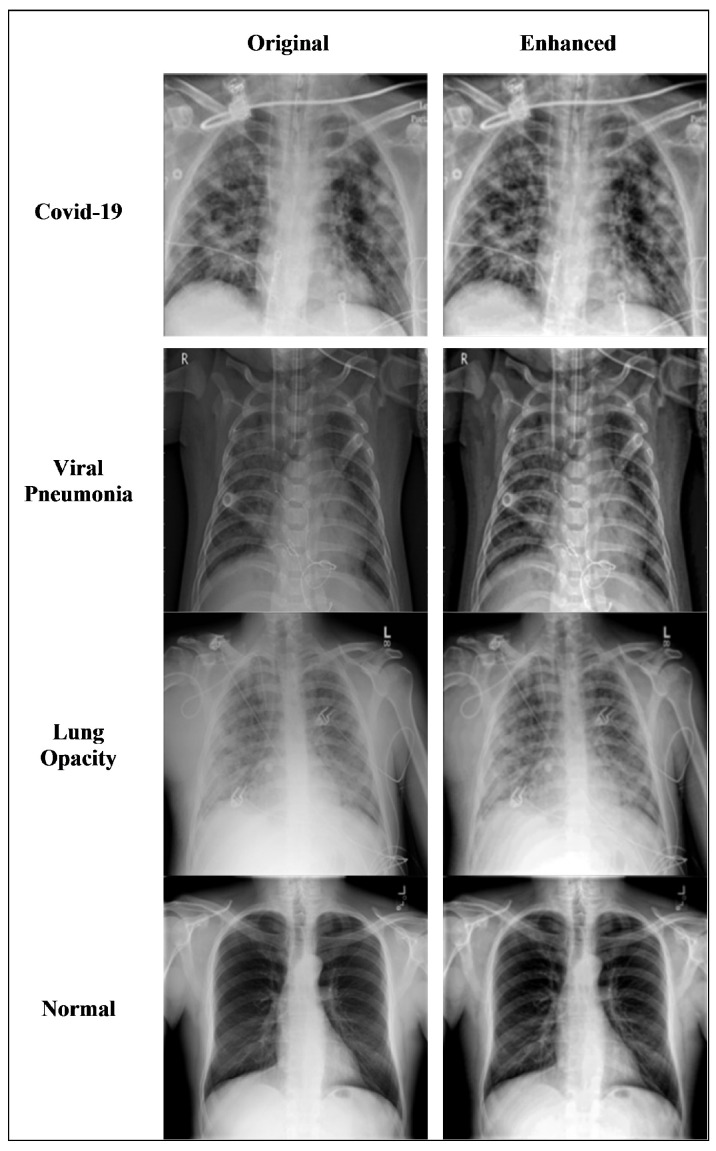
Comparison of original X-ray sample images of four classes with corresponding enhanced X-ray images.
